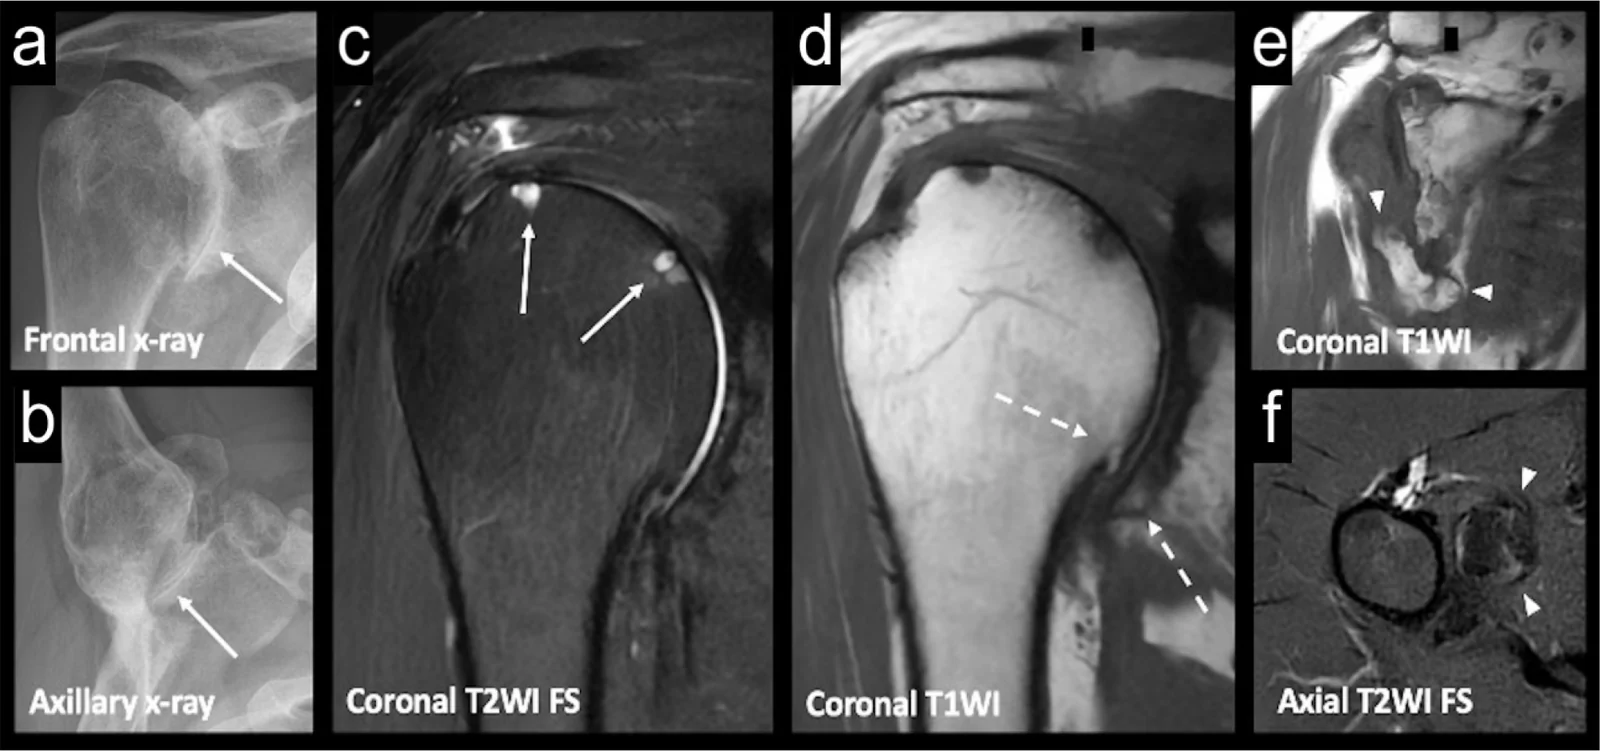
A 63-year-old male patient undergoing a post-traumatic MRI
